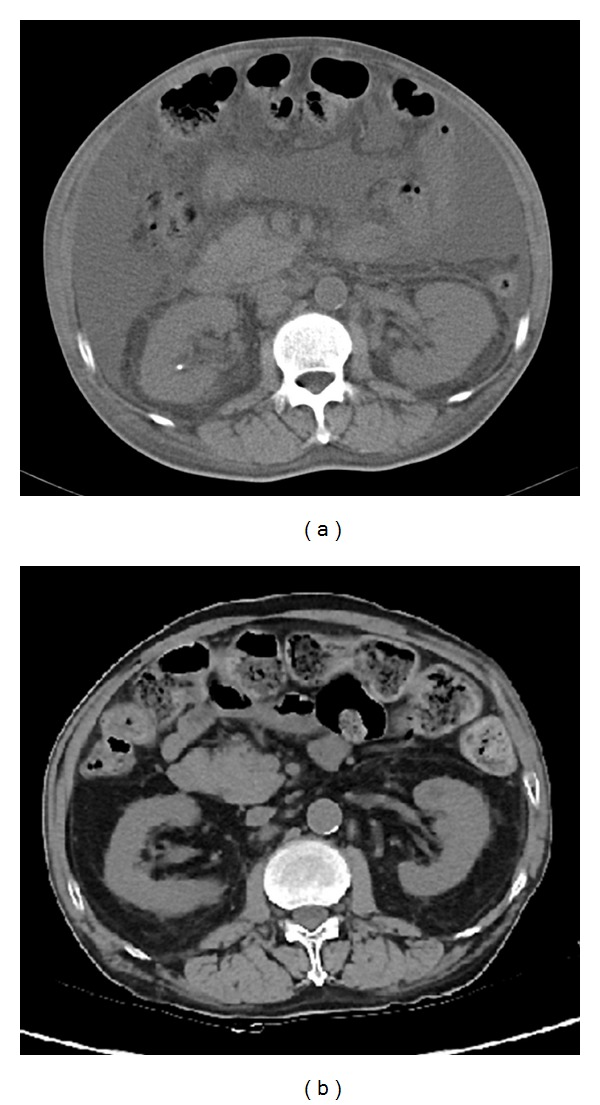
Computed tomographic scans of the abdomen. (a) Massive ascites before treatment. (b) Disappearance of ascites after treatment.
